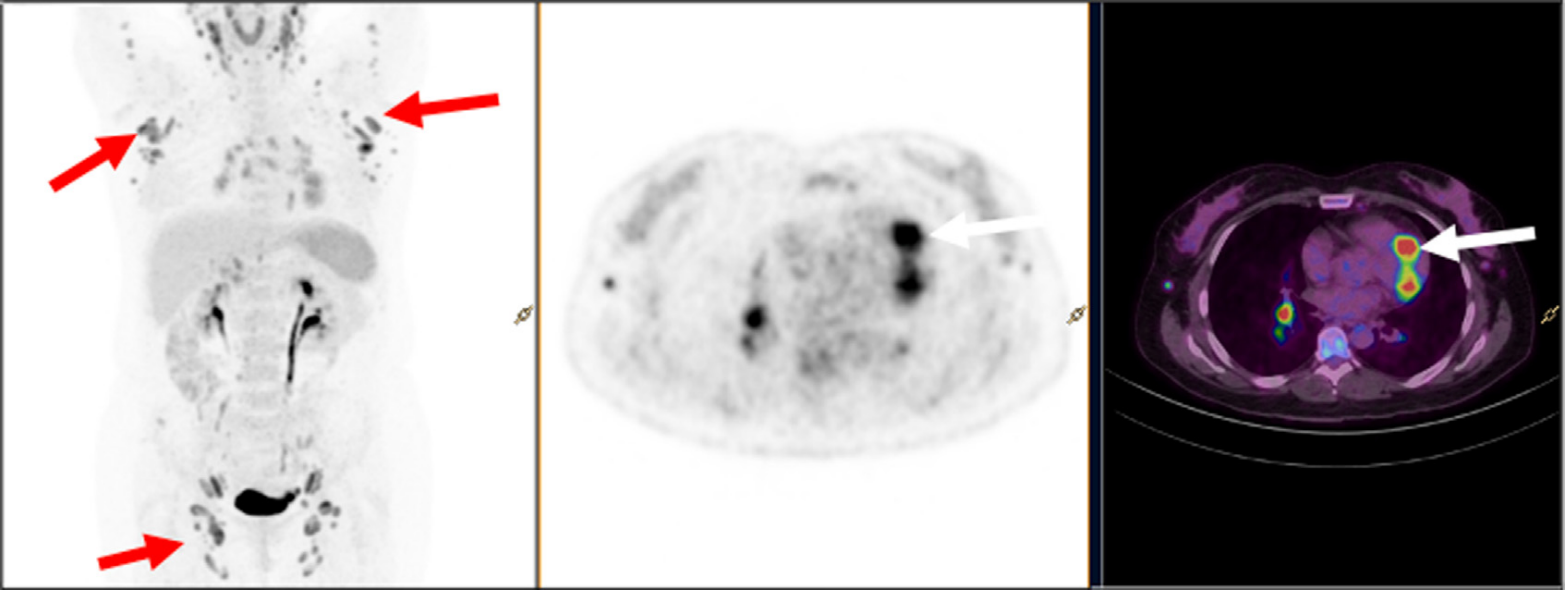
Initial Fluorine-18 Fluorodeoxyglucose Positron Emission Tomography/Computed Tomography The scan shows polyadenopathies (red arrows) and myocardial hypermetabolic foci (white arrows).
